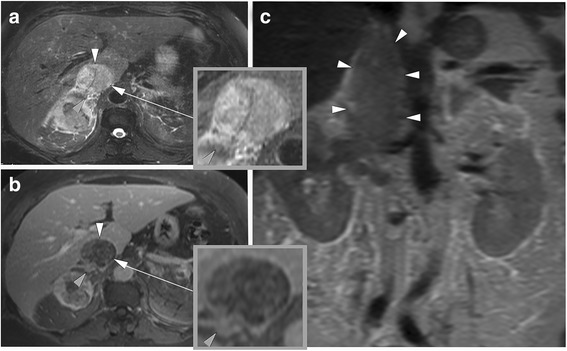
Images in a 69-year old woman with a clear cell renal cell carcinoma (RCC) and an inferior vena cava (IVC) tumor thrombus with wall invasion. The RCC extends from the right kidney into the IVC and extends into the right atrium. a axial fat-saturated T2-weighted image. b T1-weighted contrast-enhanced 3D GRE (VIBE) image contrast enhanced 3D GRE image (arterial phase) and (c), coronal T2-weighted HASTE image for anatomic reference. Note that the thrombus completely obstructs the lumen of the IVC, but also seems to breach the vessel wall (a, c). The contrast-enhanced image (b) demonstrates a heterogeneous enhancement of the tumor thrombus and a clear breach of the vessel wall (gray arrowhead), which is highly suggestive of IVC wall invasion. During extended nephrectomy, this thrombus showed strong adherence to the IVC wall and during extraction, circumferential cavectomy with vascular reconstruction became necessary. VIBE = Volumetric interpolated breath-hold examination
